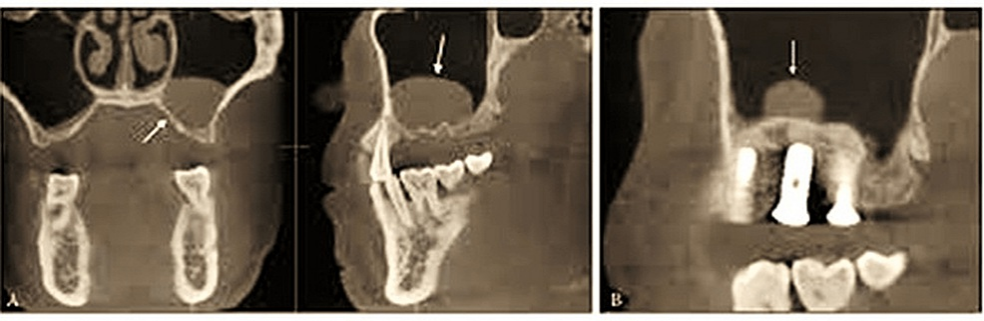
CBCT image of the region. A: sinus lift after the hydraulic system; B: bone fill. CBCT: cone-beam computed tomography
